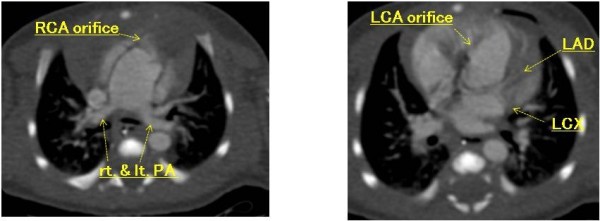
CT view. An abnormal LCA originated from the right anterior aspect and extended behind the TA.
